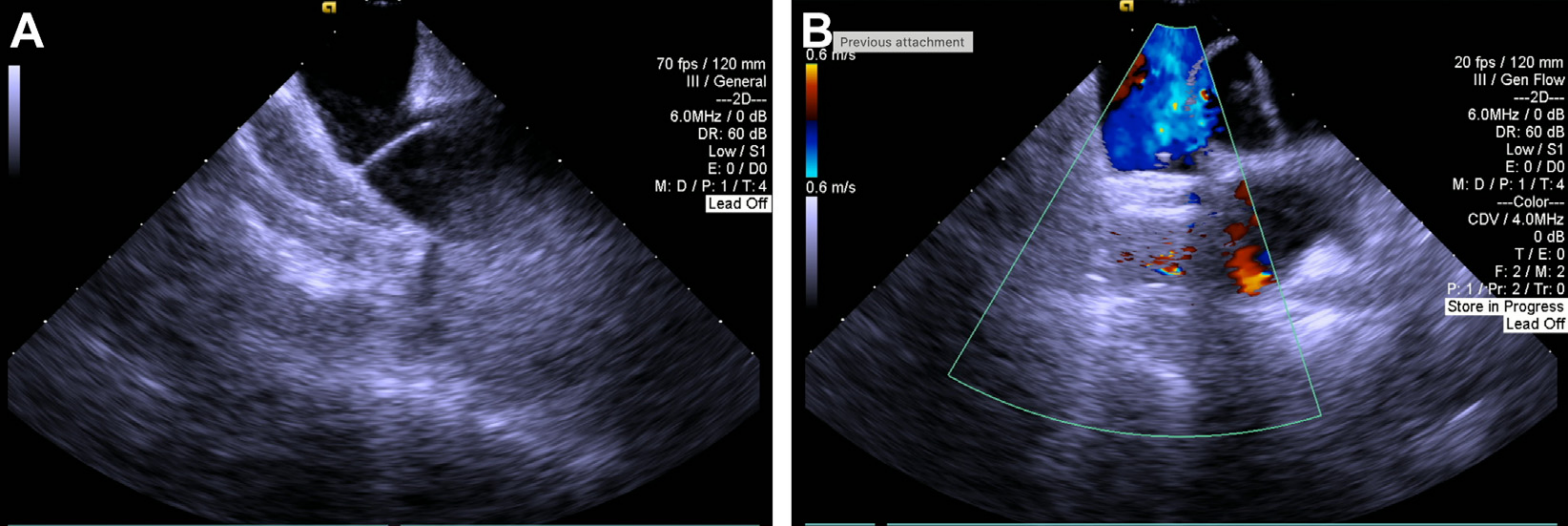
Intracardiac Echocardiography (A) Intracardiac echocardiography revealing patent foramen ovale (PFO) pre-closure. (B) Amplatzer occluder with color Doppler. Well-seated PFO closure device visualized with resolution of intra-atrial shunt.
